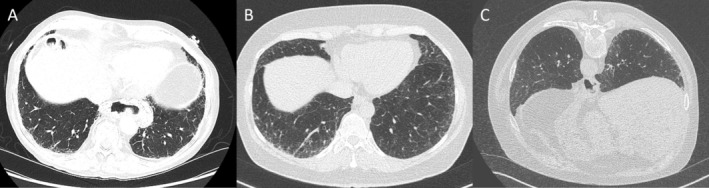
Interstitial lung abnormality (ILA) examples. (A) Subpleural, basal predominant fibrotic ILA discovered in an 88‐year‐old male undergoing evaluation for transcatheter aortic valve implantation. (B) Subpleural, basal predominant, ground glass ILA discovered in a 58‐year‐old female through CT coronary artery calcium score evaluation. (C) Subpleural, basal predominant, mixed ground‐glass and fibrotic ILA discovered in a 75‐year‐old male through CT colonography.
